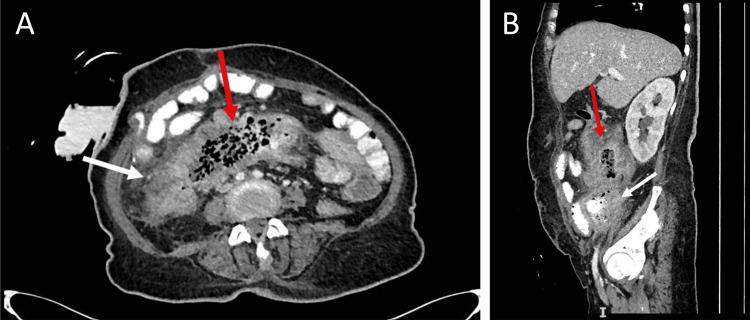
CT abdomen with oral contrast showing the abdominal collection, phelgmon, and fistulisations 1A: Cross-sectional CT image of the abdomen. 1B: Sagittal section CT image of the abdomen. Right iliac fossa phlegmon (white arrow); large mesenteric collection (red arrow) CT: computed tomography
